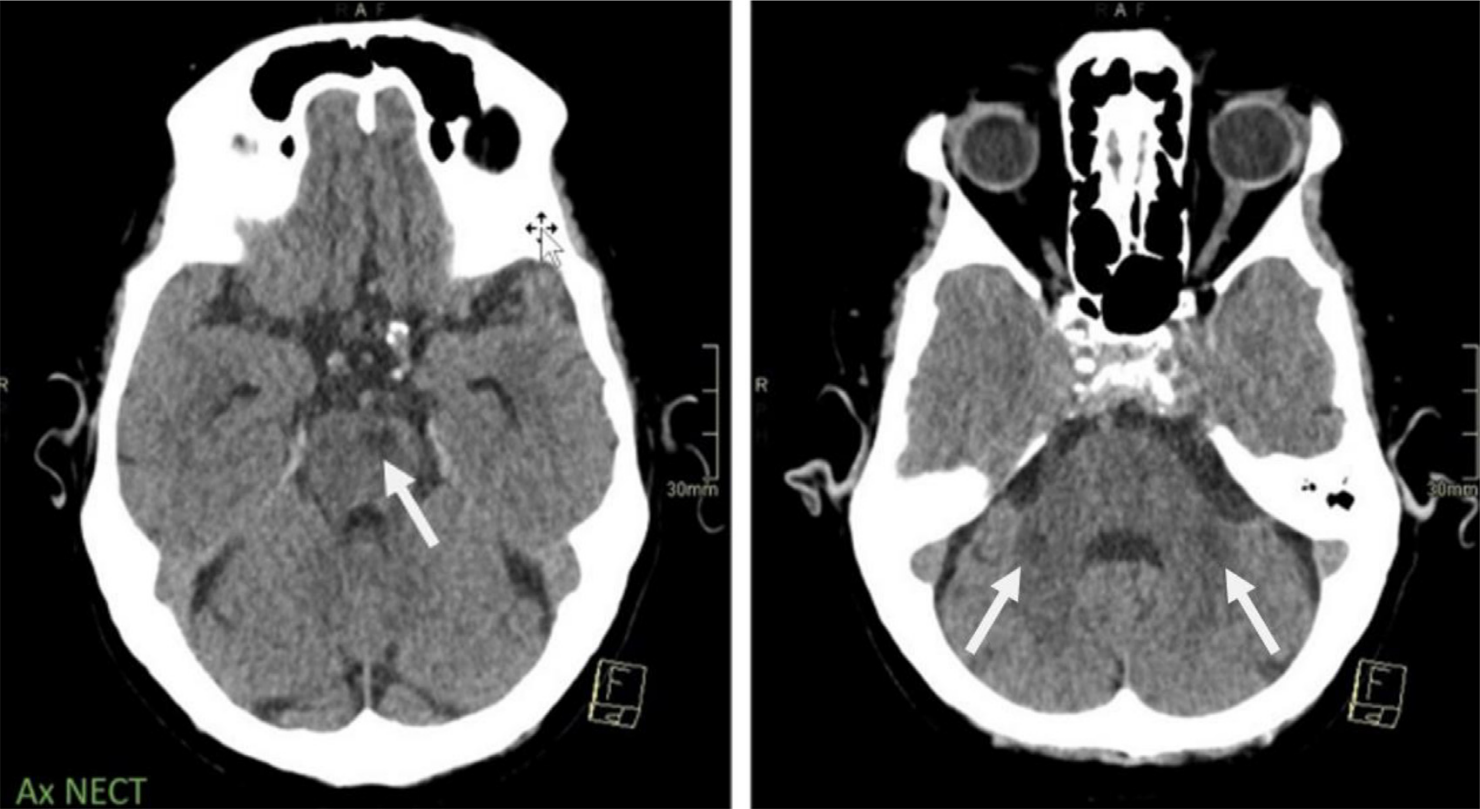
Axial noncontrast CT scan of the brain showing a small hypodensity in the left paramedian pontine region (white arrow) representing a chronic infarct (left image). Note the bilateral symmetrical hypodensities in the middle cerebellar peduncles (black arrows), consistent with Wallerian degeneration (right image).
